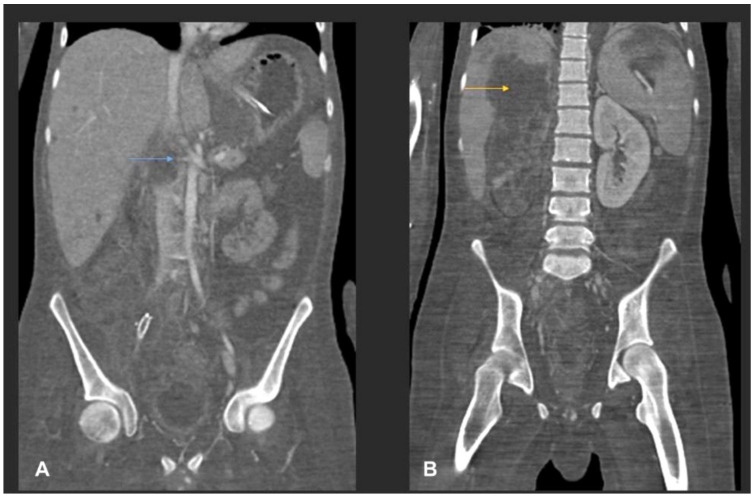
(A) MIP coronal images of abdomen shows a cut-off of the right proximal renal artery 1 cm from the ostium with adjacent inflammatory collection (blue arrow). (B) Coronal post-contrast CT shows infarction of the right kidney. A large liver abscess (yellow arrow) in the right liver lobe with extracapsular extension into the upper pole of the right kidney is also noted.
